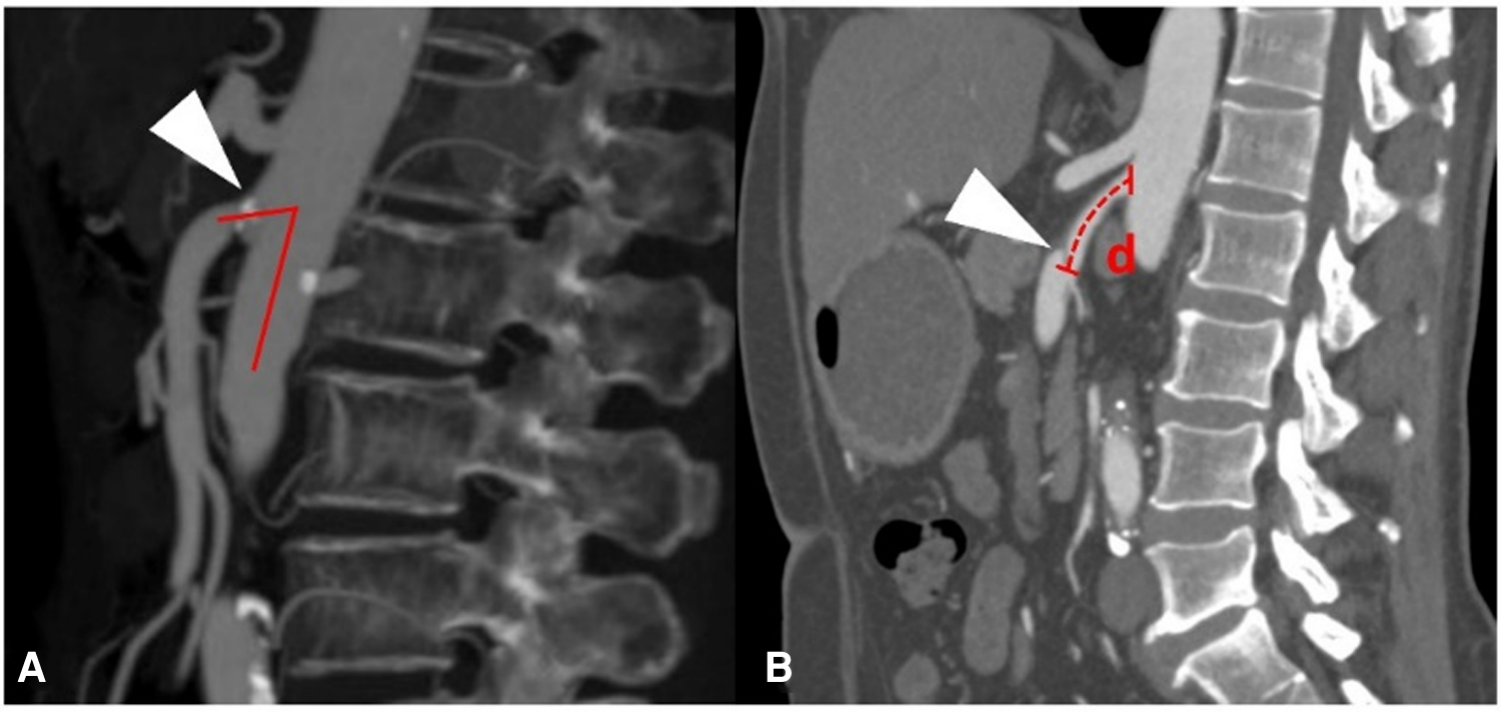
Measurements obtained using findings from computed tomography angiography. (A) Measurement of the aortomesenteric angle (red lines) and location of the superior mesenteric atherosclerotic plaque (white arrow). (B) Measurement of the distance (red *d*) from the root of the superior mesenteric artery to the origin of the superior mesenteric artery dissection (white arrow).
